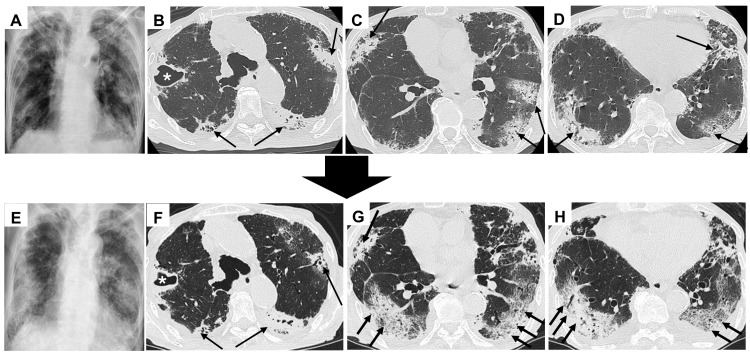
Chest images obtained from the previous hospital and our hospital (A-D) Chest images from the previous hospital. (A) Chest X-ray showing consolidations and ground-glass opacities (GGOs) in both lung fields. (B-D) High-resolution computed tomography (HRCT) images. (B) HRCT shows a cavity and granular opacities in the right upper lobe (asterisk), along with consolidation and GGOs in the bilateral upper lobes (arrows). HRCT also shows patchy consolidations and GGOs, predominantly in the lower lung peripheries (arrows). (E-H) Chest images from our hospital obtained two days after the previous imaging. (E) Chest X-ray showing extensive consolidation and GGOs in both lung fields. (F) HRCT shows a cavity and granular opacities in the right upper lobe (asterisk), consolidation, and GGOs in the bilateral upper lobes (arrows). HRCT images show more extensive consolidation and GGOs than the previous images, predominantly in patchy areas around the lower lobes of both lungs (arrows).
